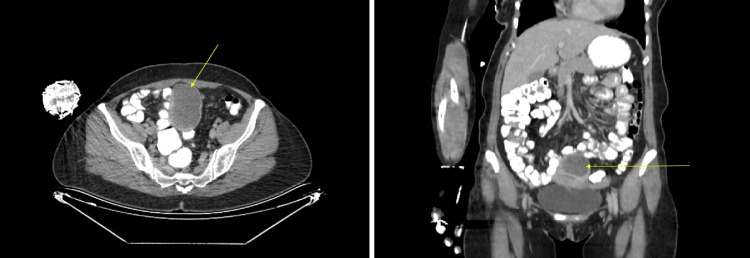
Computed tomography revealed a hypodense space-occupying lesion measuring 7.2 × 3.3 × 3.6 cm in the posterior wall of the uterine corpus (yellow arrow).
